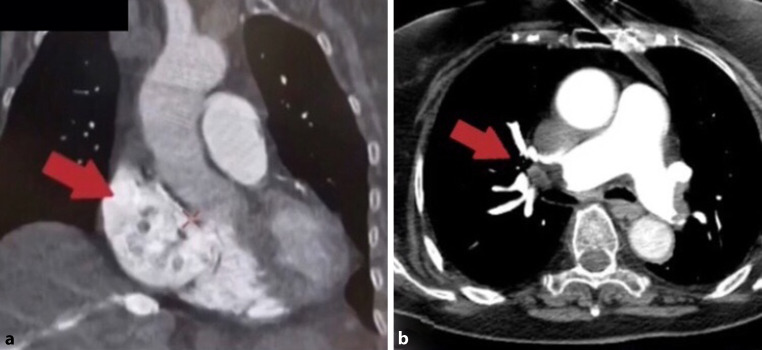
a CT pulmonary angiography (CTPA), sagittal view, depicting the presence of masses within the right atrium (*red arrow*). b CTPA, axial view, depicting bilateral pulmonary embolism (*red arrow*)
